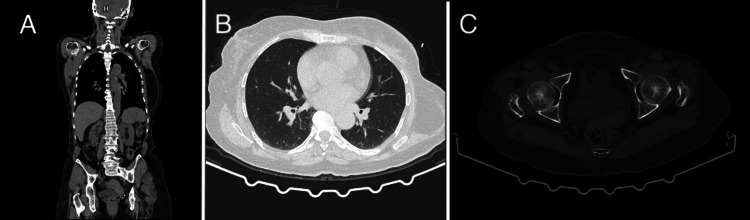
Thoraco-abdominopelvic computed tomography (CT) scan showing no evidence of distant metastatic disease in a 64-year-old patient (A) Coronal CT reconstruction demonstrating absence of detectable visceral, nodal, or osseous metastases. (B) Axial chest CT scan showing no evidence of pulmonary metastatic lesions. (C) Axial pelvic CT scan demonstrating no focal suspicious lesions.
